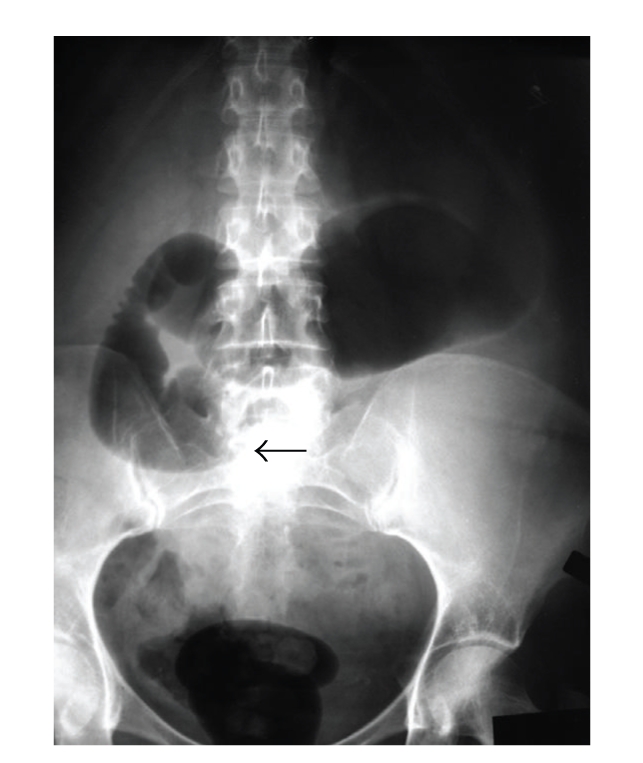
Abdominal X-ray demonstrating abrupt cut off sign at the 3rd portion of duodenum. Dilated stomach and proximal duodenum. Black arrow shows the cut-off sign.
